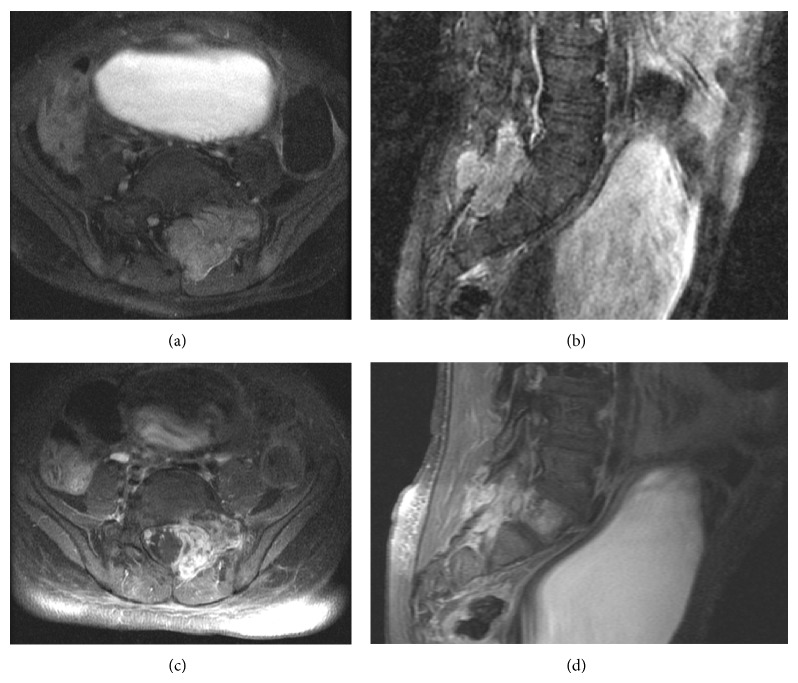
Magnetic resonance images of a sacral osteosarcoma metastasis in a 5-year-old boy. Axial (a and c) and sagittal (b and d) T1-weighted images acquired with gadolinium before treatment (a and b) and after treatment (c and d).
